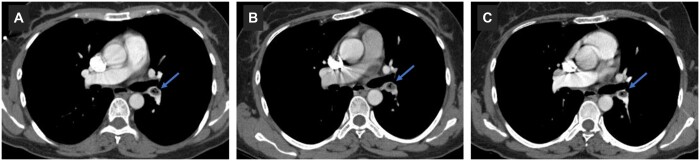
CT images at the 6th month control (A), the 1st year (B) and the 4th year (C) filling defect is indicated by arrow.
